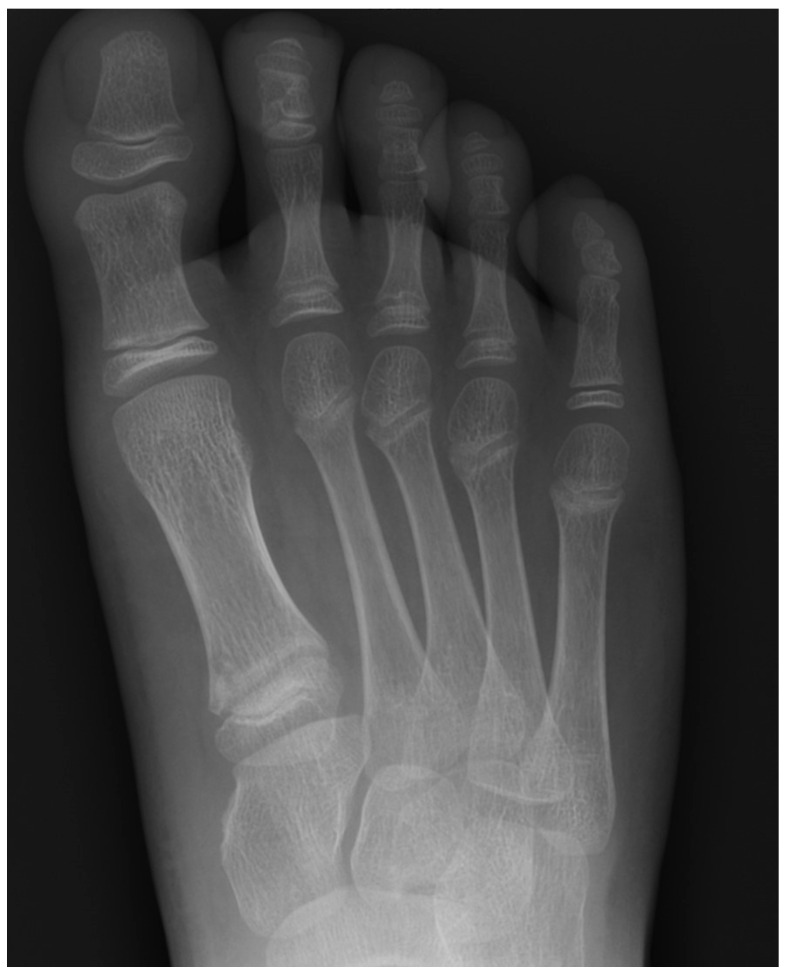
Radiograph of a 10-year-old male patient with no trauma history, showing fragmentation at the base of the first metatarsal.
